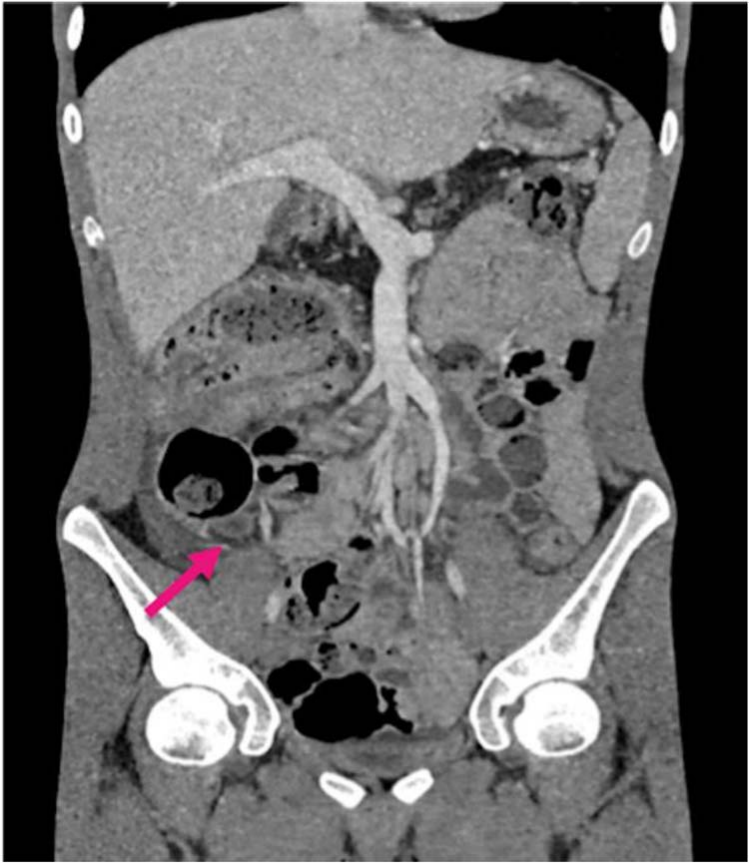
Contrast CT coronal sections depicting the classical ‘bullseye sign’ of intussusception of the caecum into the ascending colon.
